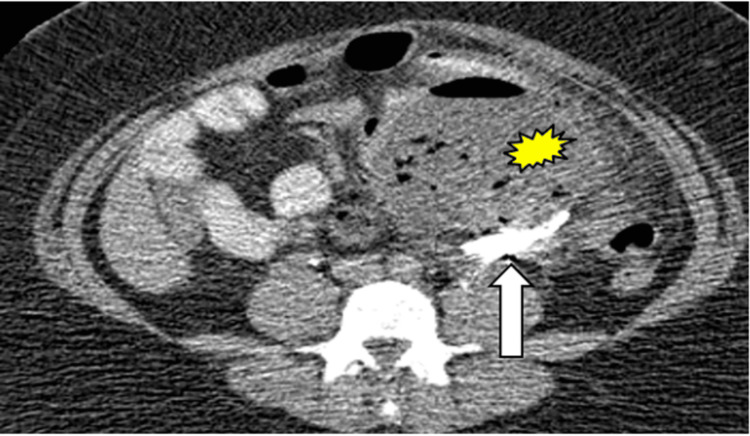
Gossypiboma. CT image shows a large intraperitoneal abscess (yellow burst) with a retained foreign body: a sponge (white arrow).
